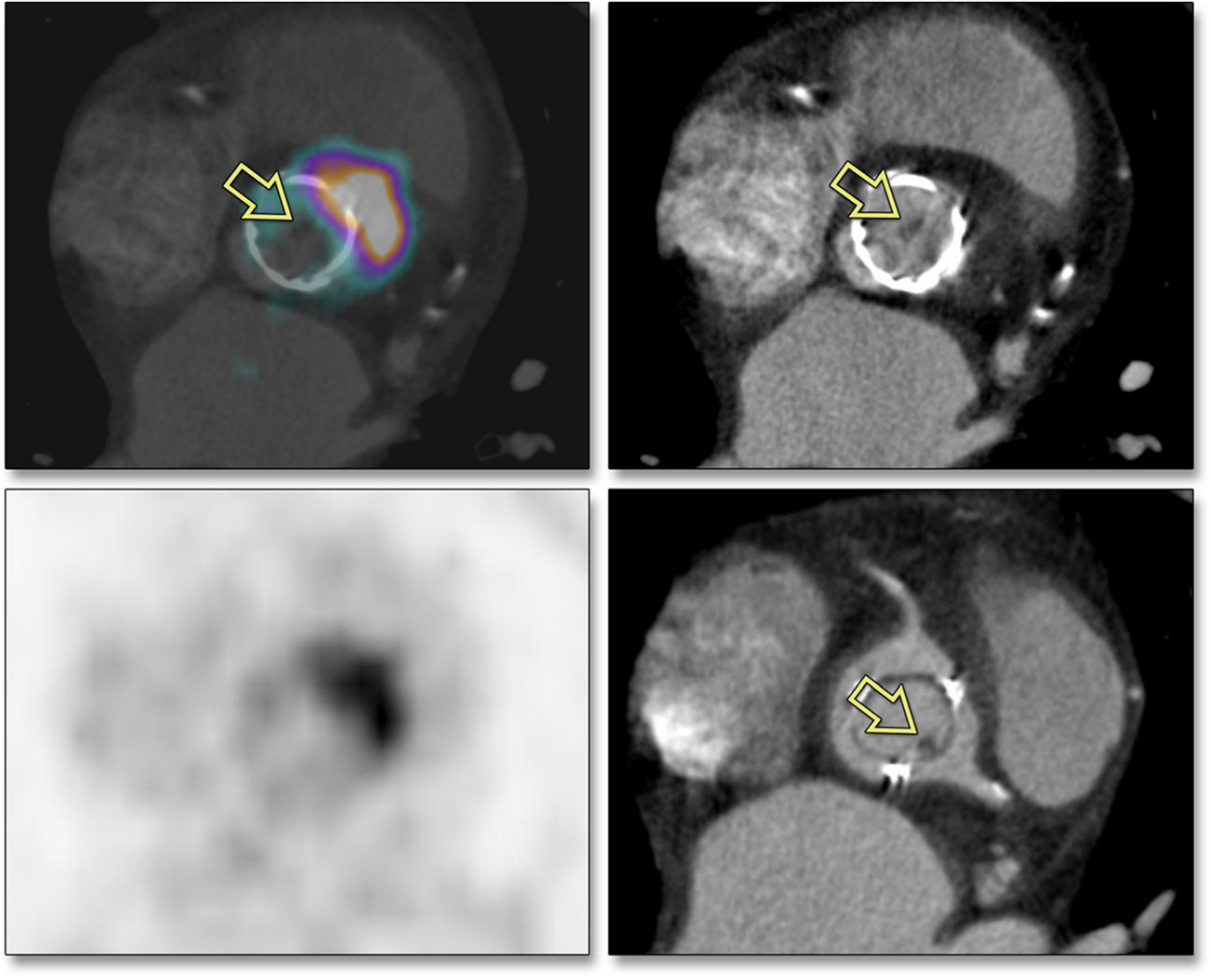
PET/CT angiography. Clinical case which demonstrates the additional value of combining FDG-PET and CTA. FDG-PET shows increased uptake at the edge of the prosthetic aortic valve, indicative of abscess formation (upper and lower left), but does not show the vegetation (*yellow arrows*) that is visible on CTA (diastolic phase: upper right; systolic phase: lower right). (This figure was published in the *Journal of the American College of Cardiology: Cardiovascular Imaging*, Vol 9, Scholtens AM, Swart LE, Verberne HJ, Tanis W, Lam MG, Budde RP, Confounders in FDG-PET/CT Imaging of Suspected Prosthetic Valve Endocarditis, Pages 1462-1465, Copyright Elsevier [2016]) [36]
